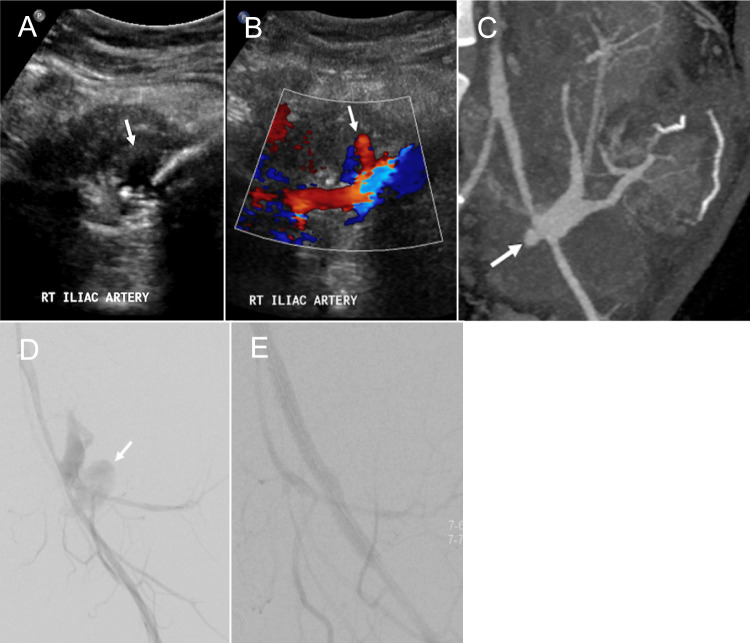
Arterial pseudoaneurysm (PSA). A 40-year-old male with a history of both type 1 and 2 diabetes mellitus and diabetic nephropathy underwent simultaneous pancreas-kidney transplantation, presenting with abdominal pain on postoperative day 40. Grayscale ultrasound (A) shows an anechoic cystic structure (arrow). Color Doppler ultrasound (B) reveals a characteristic Yin-Yang flow pattern (arrow) within the anechoic cystic structure, confirming the findings of PSA. CT angiography (C) demonstrates a saccular outpouching with enhancement (arrow). Angiography (F) confirms the outpouching filling with contrast due to the pseudoaneurysm (arrow) at the anastomotic site. Post-treatment angiography (E) demonstrates successful exclusion of the PSA following stent graft placement
